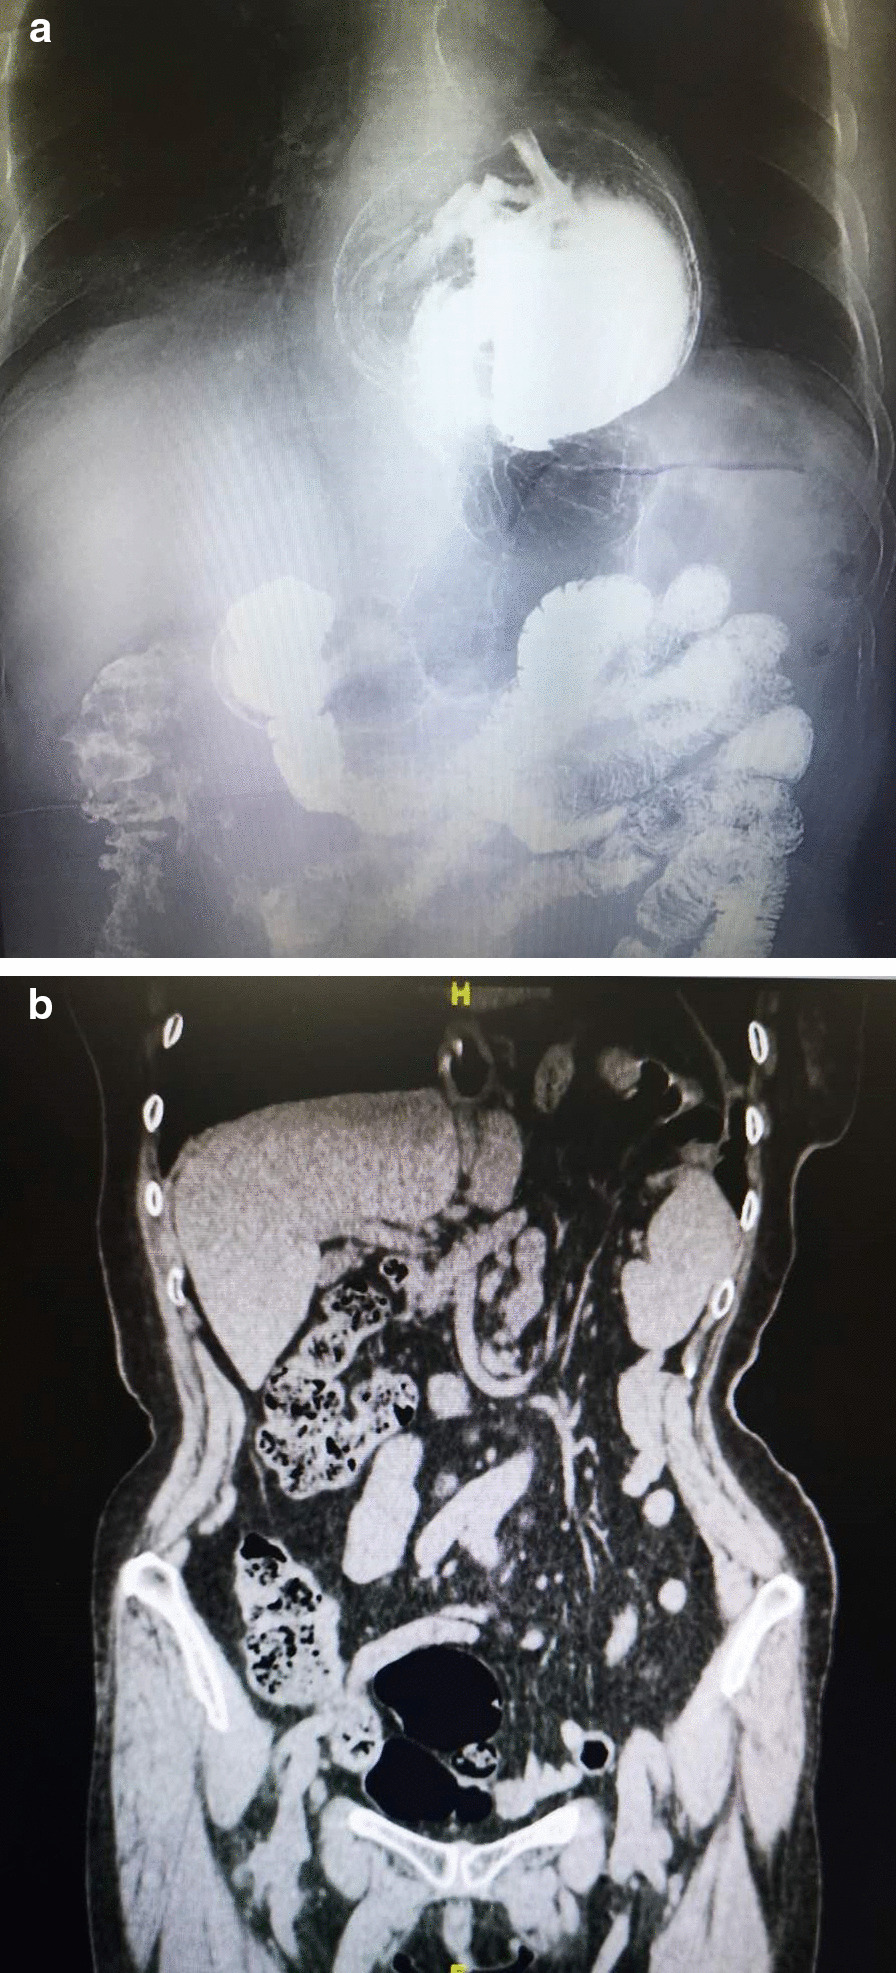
a, b Preoperative Upper GI study and CT scan showing a giant hiatal hernia
